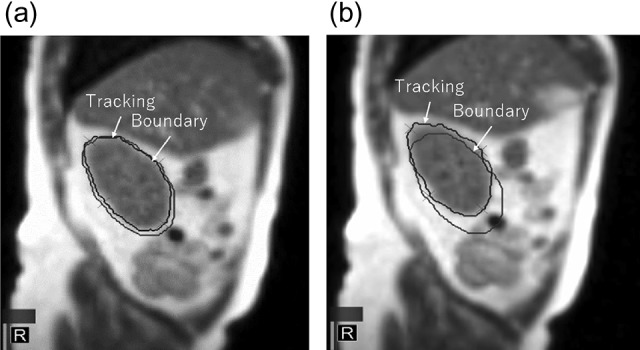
Tracking and boundary region in Cine-MR image. The tracking volume is (a) within the boundary (beam-on) and (b) beyond the boundary (beam-off).
